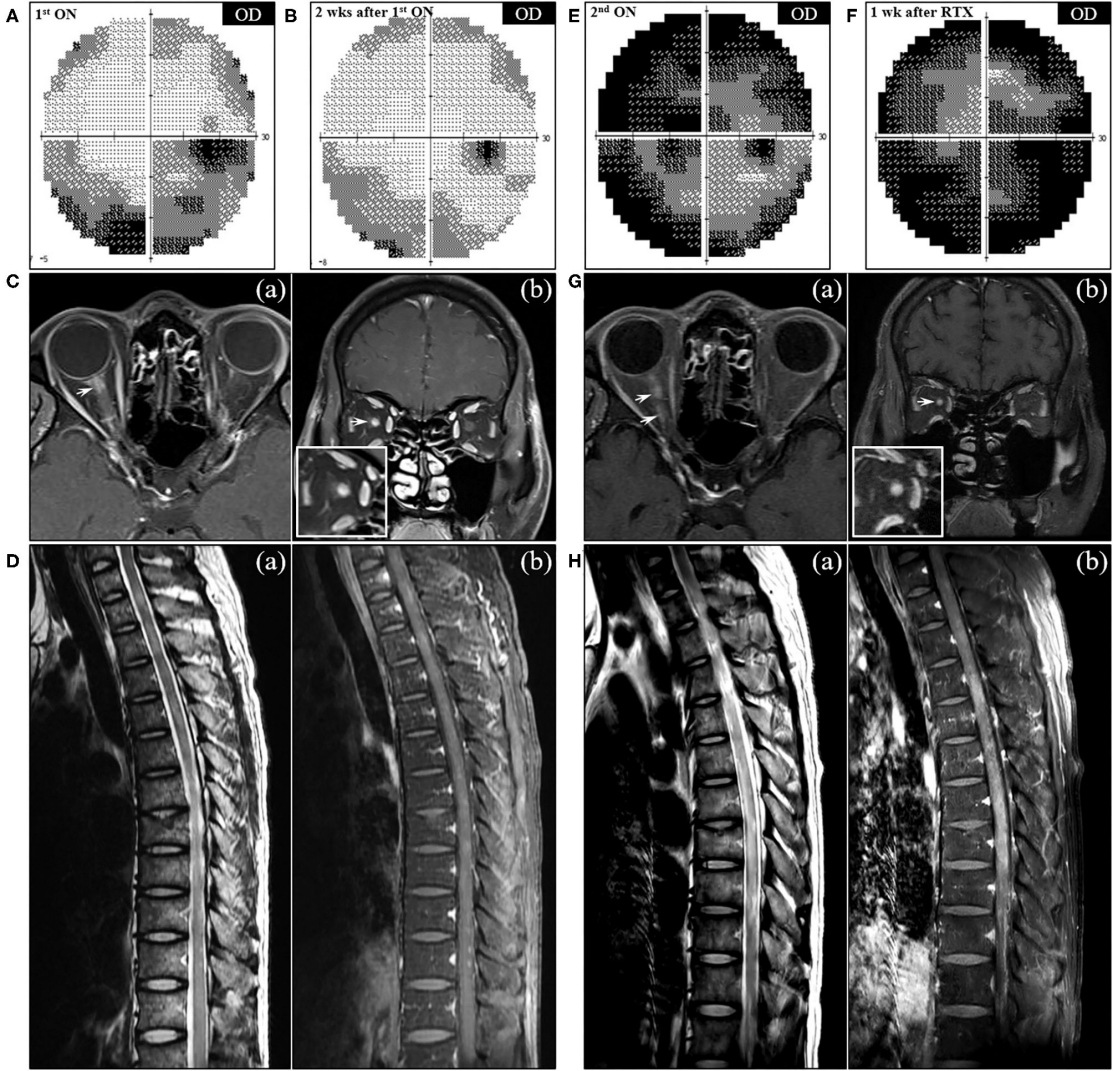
Serial visual field tests and MRI findings of NMO in the patient. (A) The initial visual field (VF) test demonstrated the incomplete loss of the inferior and peripheral vision in the right eye, which partially improved after corticosteroid therapy (B). (C) Axial (a) and coronal (b) T1-weighted orbit MR images showed contrast enhancement in the right optic nerve at the back of the eye (arrow). (D) Sagittal MRI of the thoracic spine showed multifocal T2-hyperintensity (a) with patchy contrast enhancement (b). (E) VF test performed at the second attack of ON showed near-total blindness of the right eye, which was exacerbated to complete blindness a week after rituximab injection (F). (G) Follow-up orbit MRI showed the contrast-enhancing lesions (arrows) affecting the entire right optic nerve on the axial (a) and coronal (b) T1-weighted images. (H) Follow-up spine MRI after the second rituximab injection showed long segmental T2-hyperintensity (a) with diffuse enhancement (b) in the thoracic cord extending from T4 to T11 vertebral levels. OD, oculus dexter.
